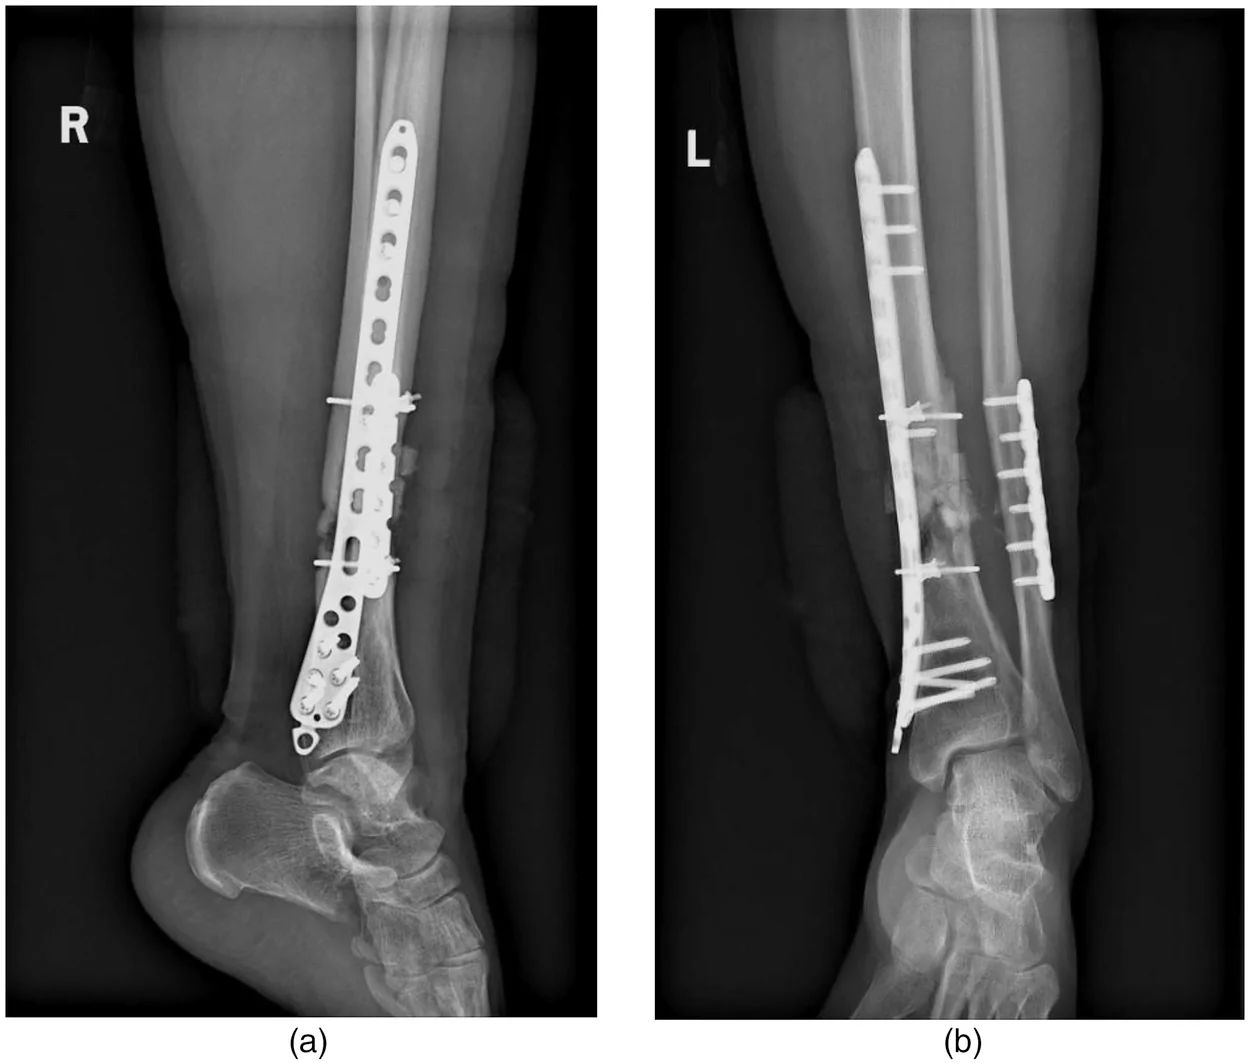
(a,b) on postoperative follow-up x-ray.
